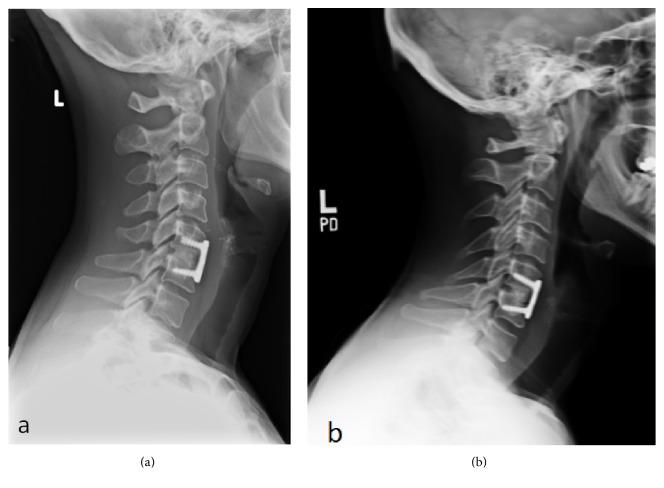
Six months postoperative X-ray images showing fusion for 1-level ACDF treated using (a) frozen allograft and (b) glycerol-preserved allograft.
